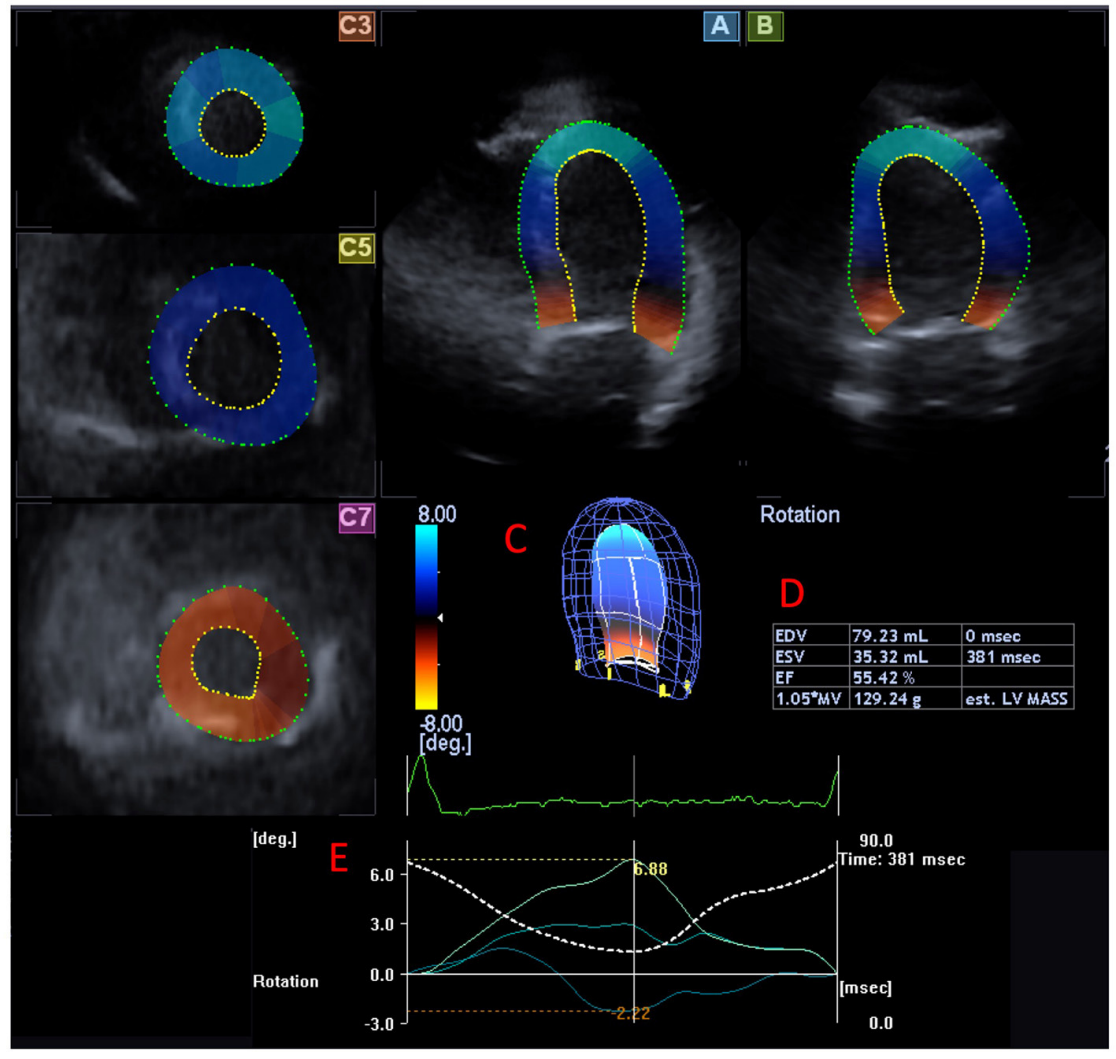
Three-dimensional (3D) speckle-tracking measurement of left ventricular (LV) rotational mechanics from an LV focused view in a patient with hypopituitarism is presented. Apical four-chamber (A) and two-chamber views (B) and apical (C3), midventricular (C5), and basal (C7) short-axis views are presented extracted from the acquired 3D volumetric dataset are presented. (C) Shows the software-generated LV cast, while (D) presents LV volumetric parameters. (E) Section shows the LV rotation curves and corresponding peak values at the apical, midventricular, and basal LV levels (colored lines). Dashed line represents LV volumetric changes during the cardiac cycle.
